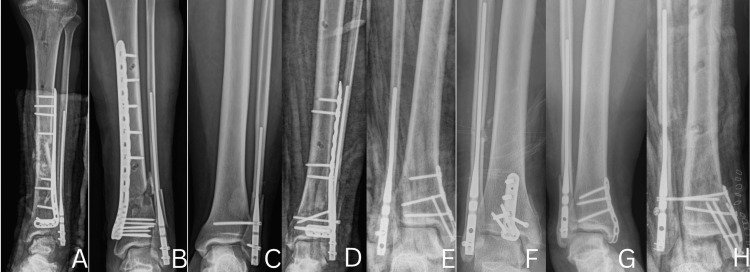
Immediate postoperative radiographs of patients following fibular nail fixation This figure is original and derived from data collected in the present study. The alphabetical identifiers (e.g., A, B, C, etc.) used in the figure are arbitrary and were created solely for the purpose of referencing specific cases within this article. These identifiers do not correspond to any patient-identifying information.
